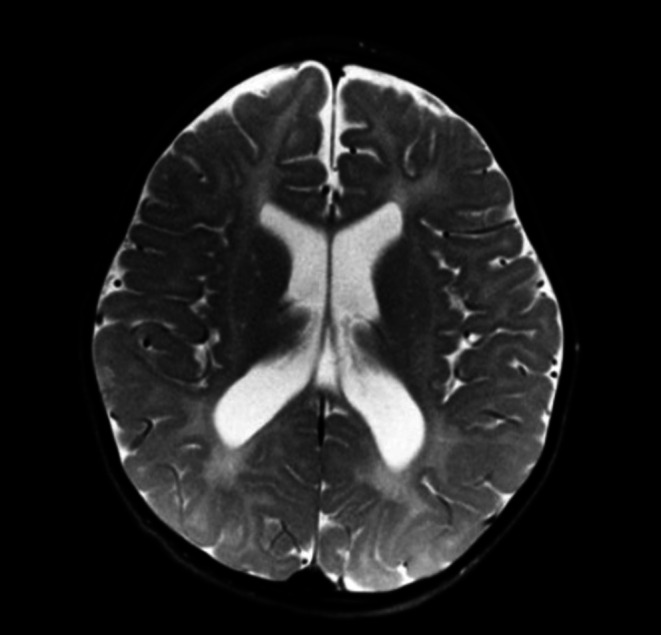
Brain MRI at five and half years of age showing significant hypomyelination in T2‐weighted axial image.
